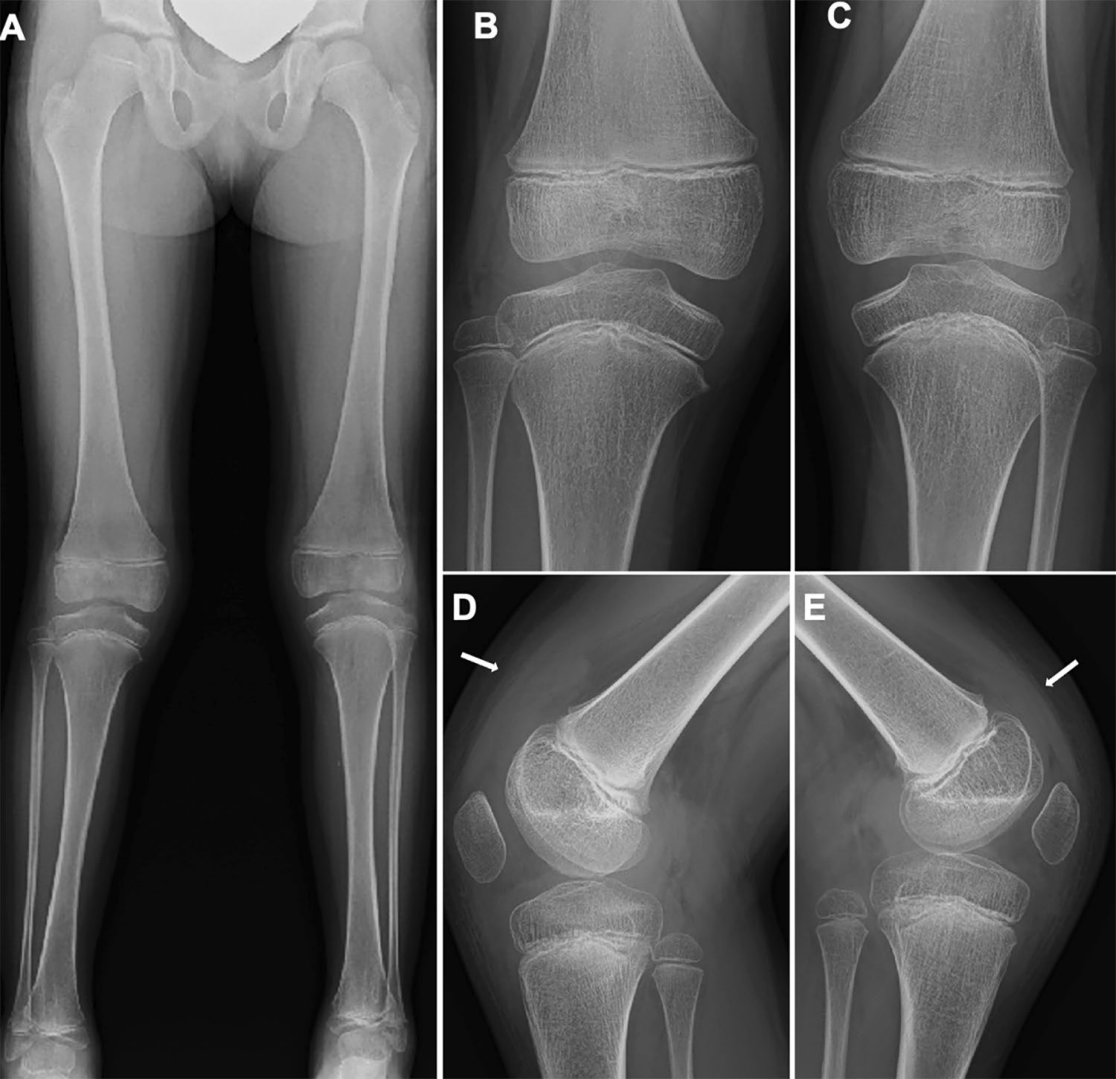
Oligoarticular type JIA. A–E. A 6-year-old girl with intermittent pain of bilateral knee joints that started at age 2 years, but remained undiagnosed until age 6 years. The frontal radiograph of the lower extremities showed leg length discrepancy with right genu valgum (A). The frontal radiograph of the knees showed large, squared epiphyses and the metaphyseal broadening of both knees. The tibial growth plates show upward convexity instead of a normal horizontal direction (B, C). The lateral radiograph of the knees depicted dilatation of the suprapatellar bursae of the right knee (arrow, D) and to a lesser extent of the left (arrow, E)
